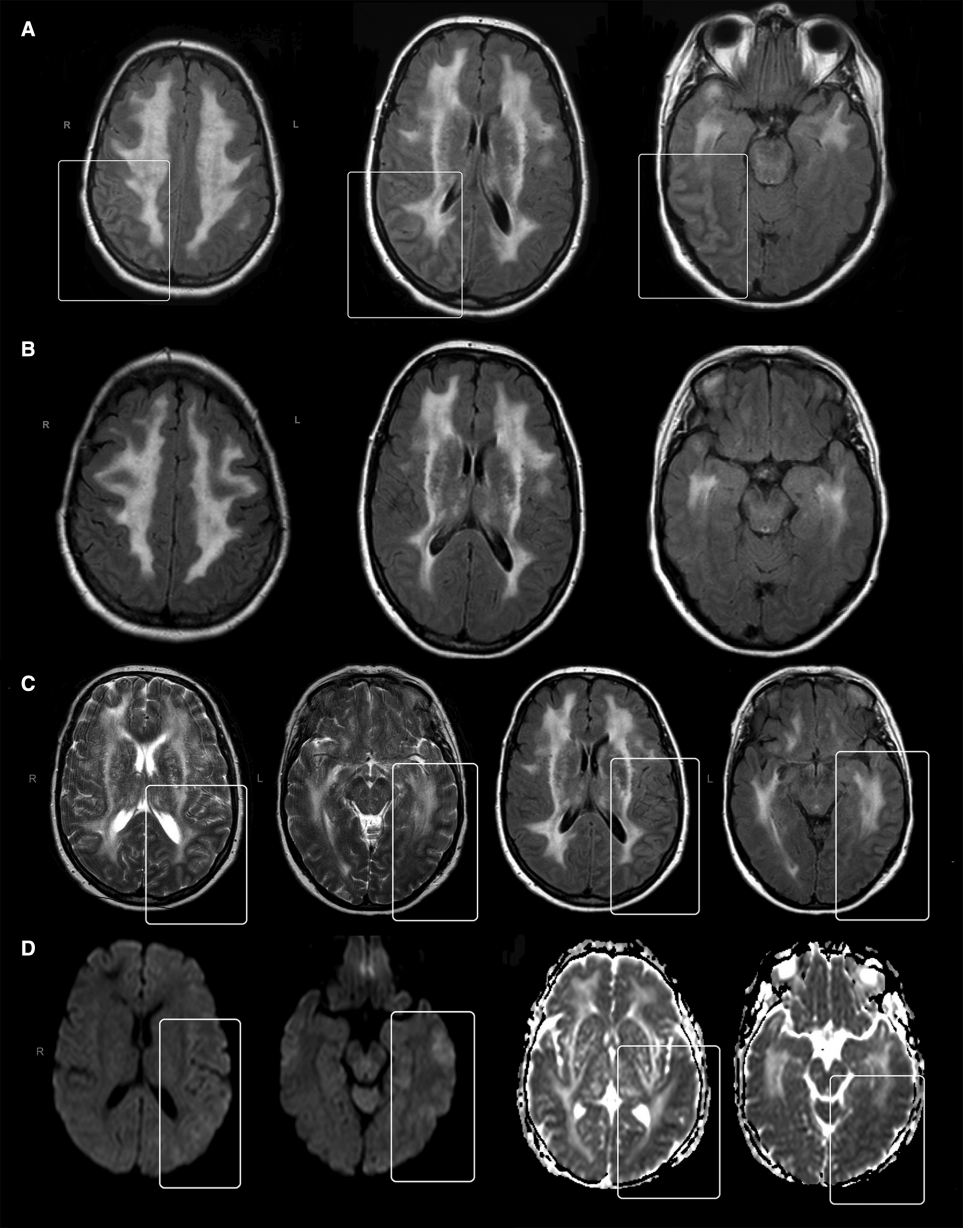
Patient 1 during first episode. Flair images revealing increased signal and swelling in the right temporal and parietal regions (approximate area of changes marked) (A) with near complete resolution of changes on follow-up images 40 days later (B). Patient 1 during the second episode. T2 weighted and flair images (C) revealing cortical swelling and signal abnormalities in the left parietal and temporal region (marked area). Diffusion-weighted image (DWI) images are showing mild increase in signal with corresponding low signal on apparent diffusion coefficient map (D).
